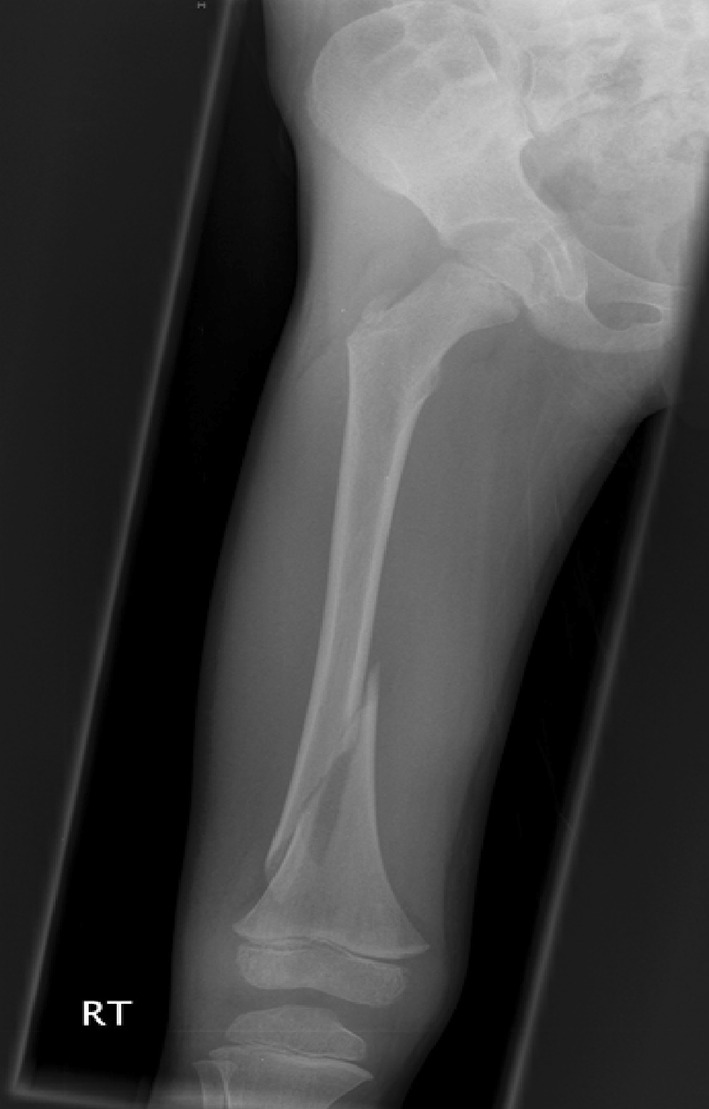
AP radiograph demonstrating a distal 1/3 spiral femoral diaphyseal fracture in a 6-year-old child
